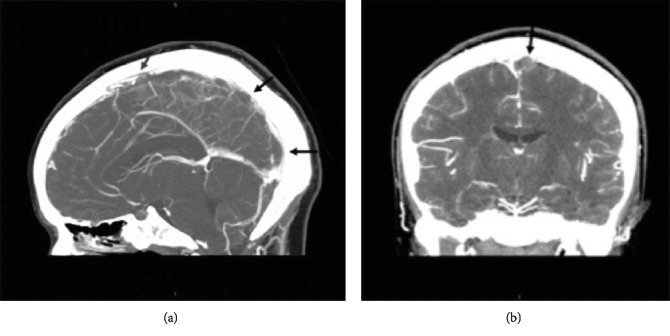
(a) CT venogram with sagittal images with filling defects of the superior sagittal sinus (arrows). (b) Coronal images with filling defects of the superior sagittal sinus (arrow).
